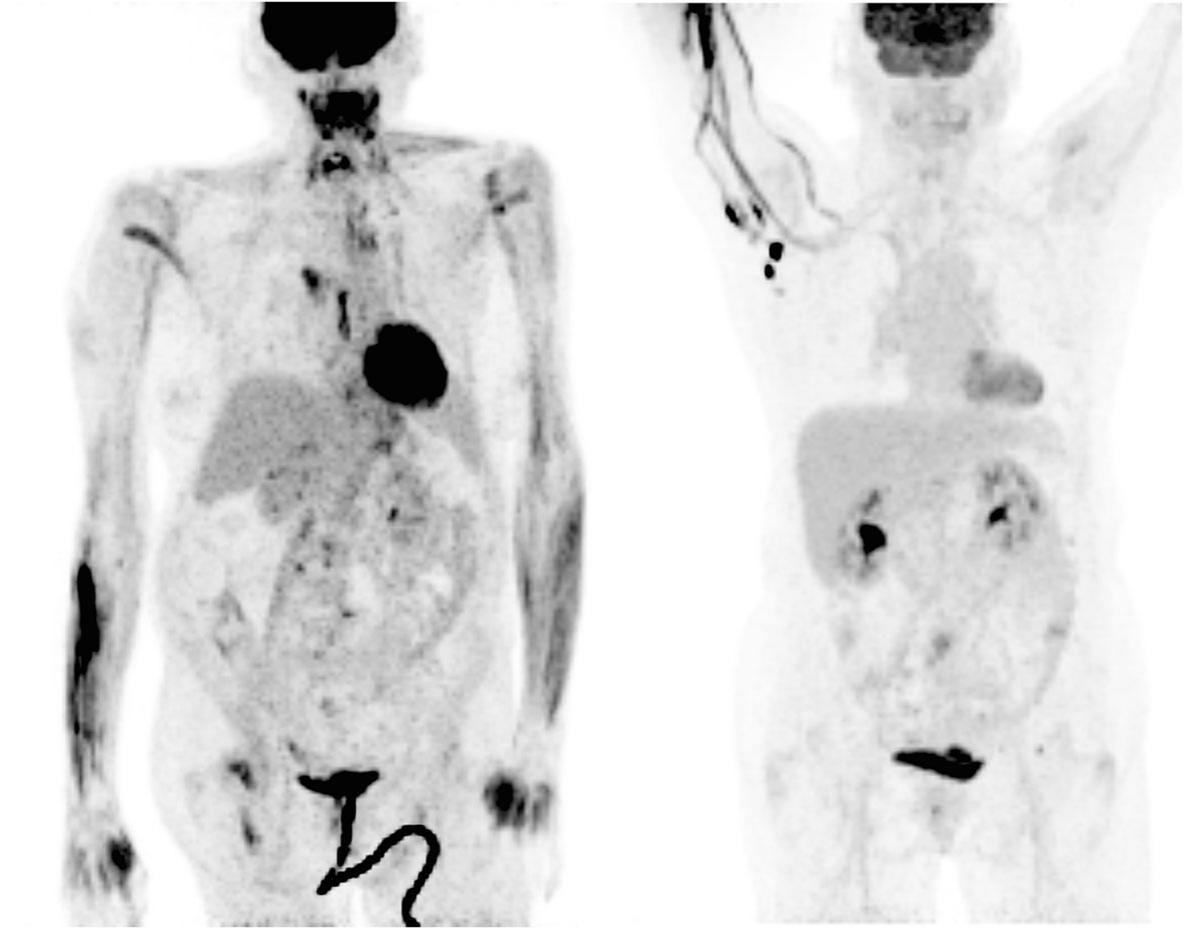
shows two examples of extravasated doses. The left image shows a visible infiltration with abnormal FDG distributions and high image noise related to reduced counts distributed through the patient. The right image shows the infiltration visible in the arm with high nodal uptake that was later determined to only be related to infiltration of the PET tracer dose
